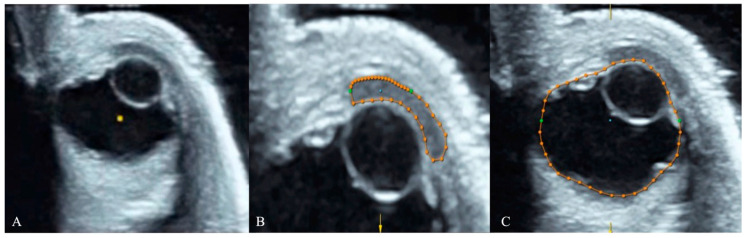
Three-dimensional postmortem ultrasound (3D PM-US) of the fetal orbit (A) showing multiplanar VOCAL™ analysis for quantitative volumetry of the cornea (B) and the eyeball (C). The contours were manually traced in six rotational planes spaced 30° apart to compute corneal and ocular volumes for ratio calculation.
